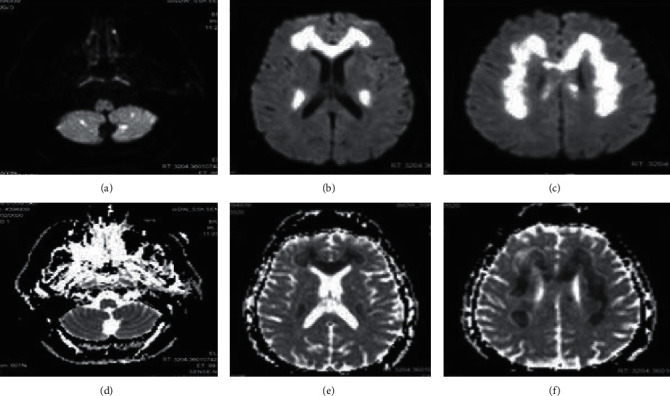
DWI brain MRI showing a hypersignal in the cerebellar hemisphere (a), corpus callosum (b, c), and corona radiata (c). There was a corresponding decrease of ADC values on the ADC map (d–f).
